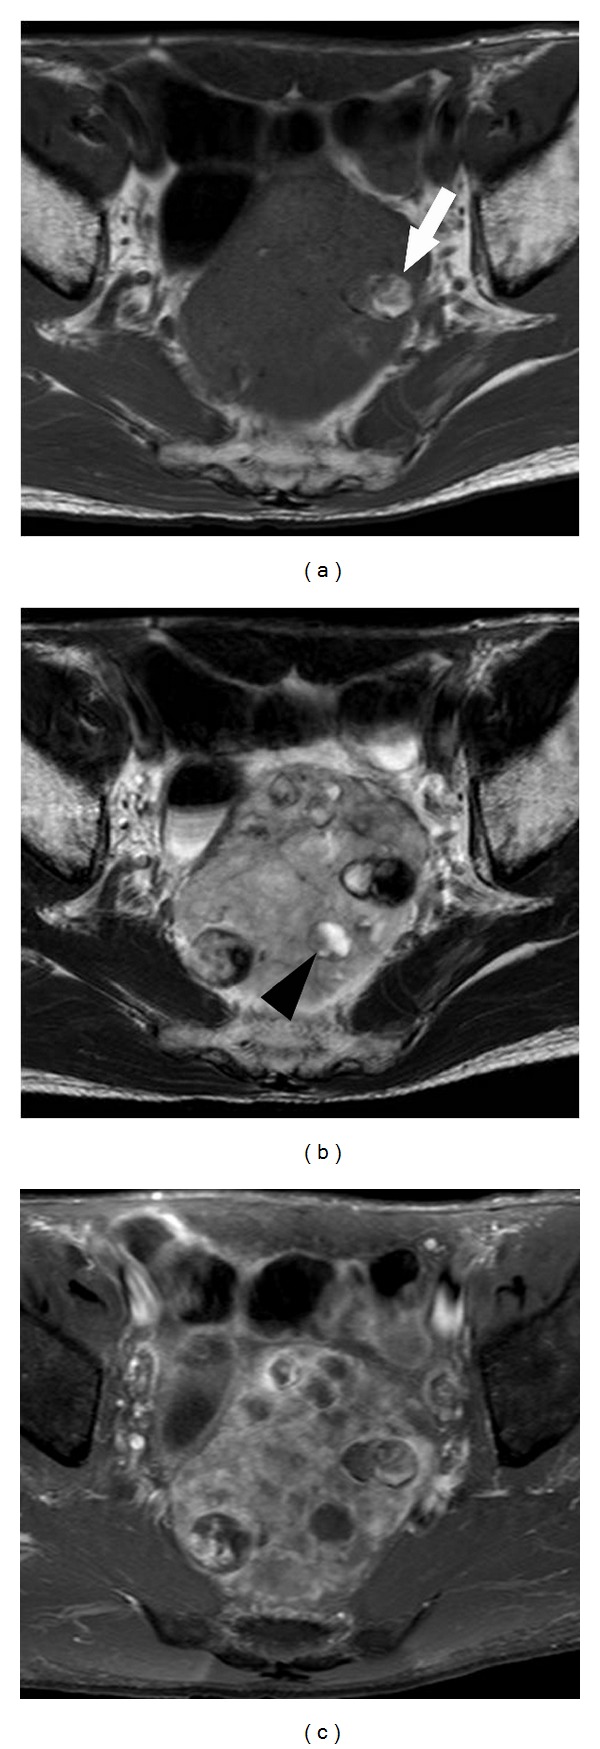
(a) T1-weighted MR image shows a well-defined hypointensity mass with focal high-intensity area (arrow). (b) T2-weighted image shows heterogeneous hyperintensity mass with spotty hyperintensity area (arrowhead). (c) Contrast-enhanced image shows slight enhancement in solid component of the tumor.
